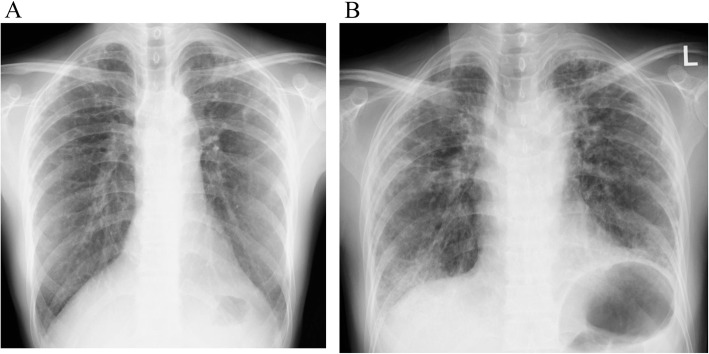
Chest radiography findings. (a) Chest radiography 3 years before admission showed apical pleural thickening and small reticular opacity (b) Chest radiography at 24 weeks of gestation showed subpleural thickening, reduced pneumatization in the upper lung, and reticular opacity throughout the lung
